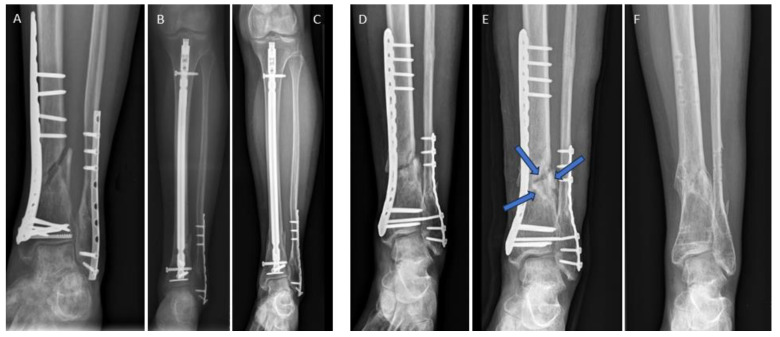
Two comparable cases with distal tibia non-union after fracture surgery. (A–C) Conventional revision surgery. (A) Radiograph showing the non-union of the distal tibia after treatment with metal plates (B) Radiograph 4 weeks post-revision with removal of tibial plate, intramedullary reaming, and insertion of a tibia nail. (C) Radiograph 24 months after revision. Bone healing was recorded after 12 weeks. (D–F) Treatment with human allogeneic cortical bone screws. (D) Non-union after osteosynthesis with metal plates. (E) Revision was performed with three Shark Screws^®^ inserted over the non-union site (blue arrows) acting as strain reducers [26]. (F) Bone healing was observed 12 weeks after revision. Plates and metal screws were removed 13 months after successful revision due to hardware-related symptoms.
